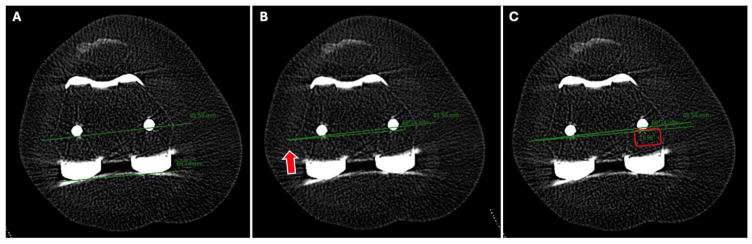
Measurement of femoral component rotation on post-operative axial CT scans. (A): One line represents the trans-epicondylar axis (TEA) and another line is drawn connecting the most posterior aspects of the femoral component (posterior condyle line). (B): The posterior condyle line is transposed (red arrow) to the TEA. (C): The angle subtended by both these lines is taken as the femoral component rotation and is highlighted here in the red box.
